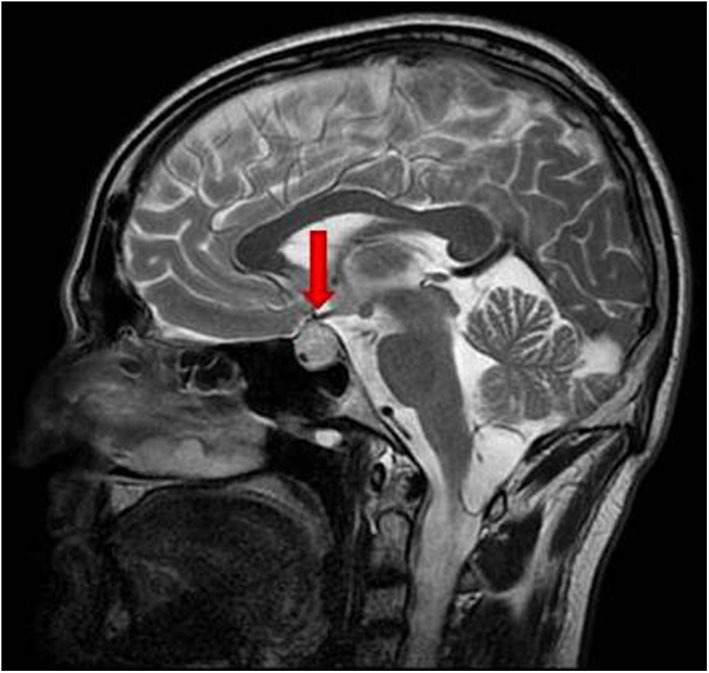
T2-weighted basal sagittal pituitary MRI performed at diagnosis. The red arrow indicates the sellar mass.
